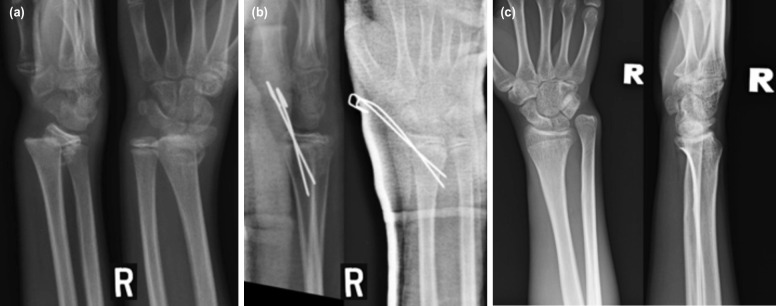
Photo showing (a) the initial fracture of the distal radial physis with dorsal displacement and angulation in one of the children in this study who was a 12-year-old boy, (b) the physeal fracture was successfully reduced both on AP and lateral views, and was immediately fixed with two smooth K wires to maintain the reduction, and (c) at skeletal maturity, the physeal arrest of the distal radius was observed on plain radiographs manifested by reduction in radial height compared to the ulna on AP view and loss of normal metaphyseal contour of the distal radius subarticularly on lateral view. The metaphysis immediately under the wrist joint appeared short and deformed on lateral radiograph. Despite this, the child had good overall result at skeletal maturity.
